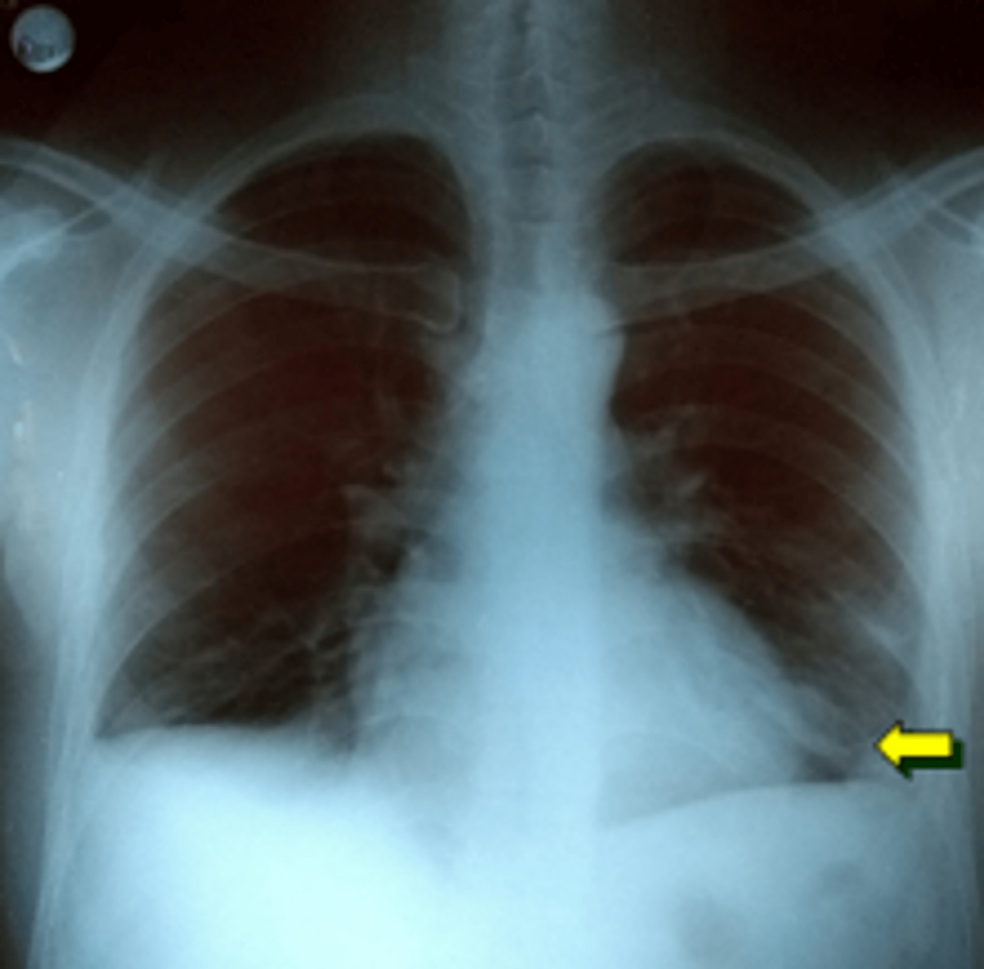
Chest radiograph revealed a heterogeneous, cavitated lesion in the left hemithorax (positive radiologic Felson sign)
